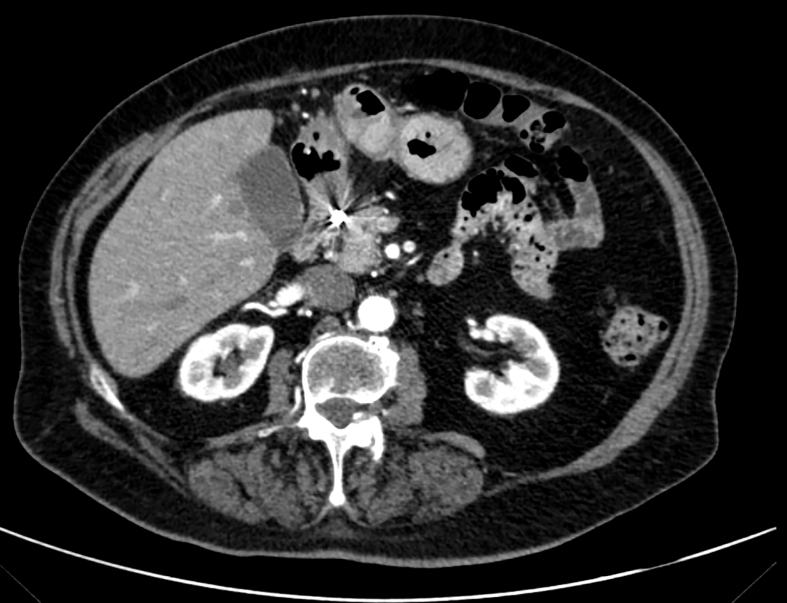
CT scan showing complete obliteration of the pseudoaneurysm of the gastroduodenal artery, with no residual sign of replenishment.
